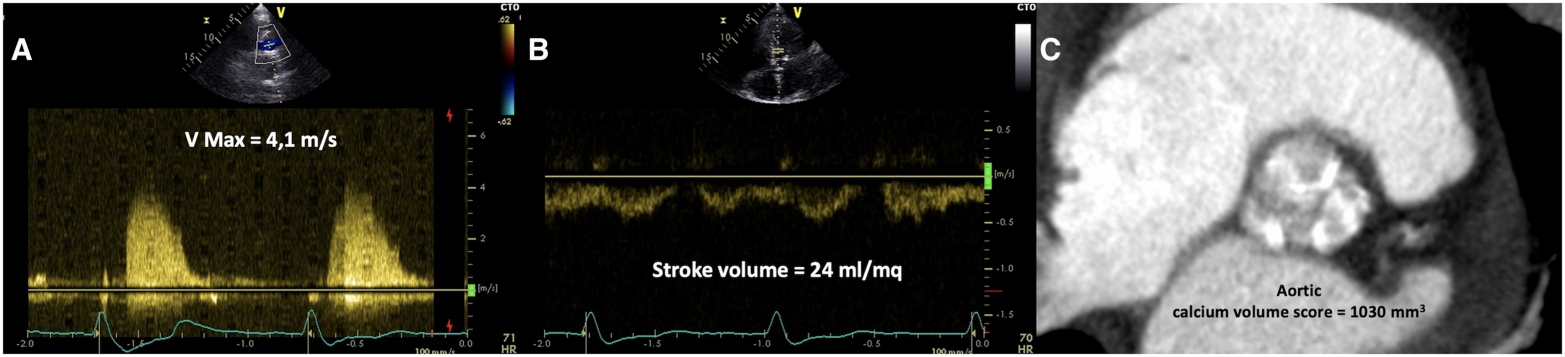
(A) TTE continuous wave Doppler on right parasternal view showing peak aortic velocity of 4.1 m/s. (B) TTE pulsed‐wave Doppler on left ventricle outflow tract evidencing an indexed stroke volume of 24 mL/m^2^. (C) Contrast‐enhanced multi‐slice computed tomography short‐axis view showing the aortic valve characterized by a high calcium volume (1030 mm^3^). TTE, transthoracic echocardiography.
